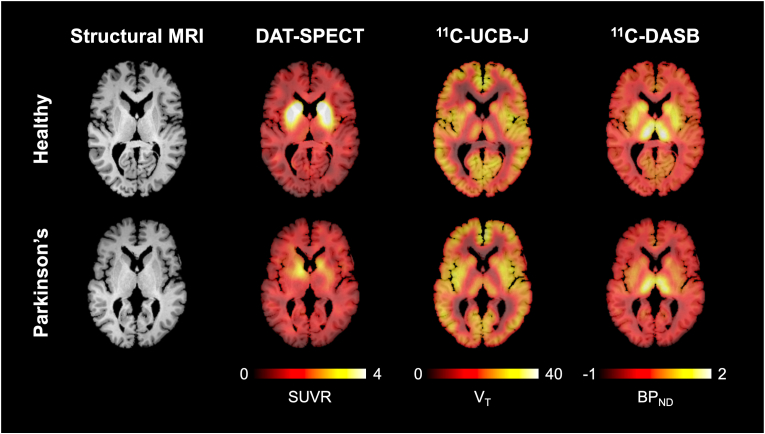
Representative imaging examples from a healthy control (55-year-old male) and an idiopathic Parkinson's disease patient (56-year-old male). From left to right, axial slices of brain extracted structural 3D T1-weighted MPRAGE magnetic resonance imaging (MRI), ^123^I-FP-CIT dopamine transporter (DAT) single-photon emission computed tomography (SPECT) standardised uptake value ratio (SUVR), ^11^C-UCB-J positron emission tomography (PET) total volume of distribution (V_T_), and ^11^C-DASB PET binding potential (BP_ND_) parametric maps. Molecular imaging parametric maps are overlaid on the corresponding structural MRI.
